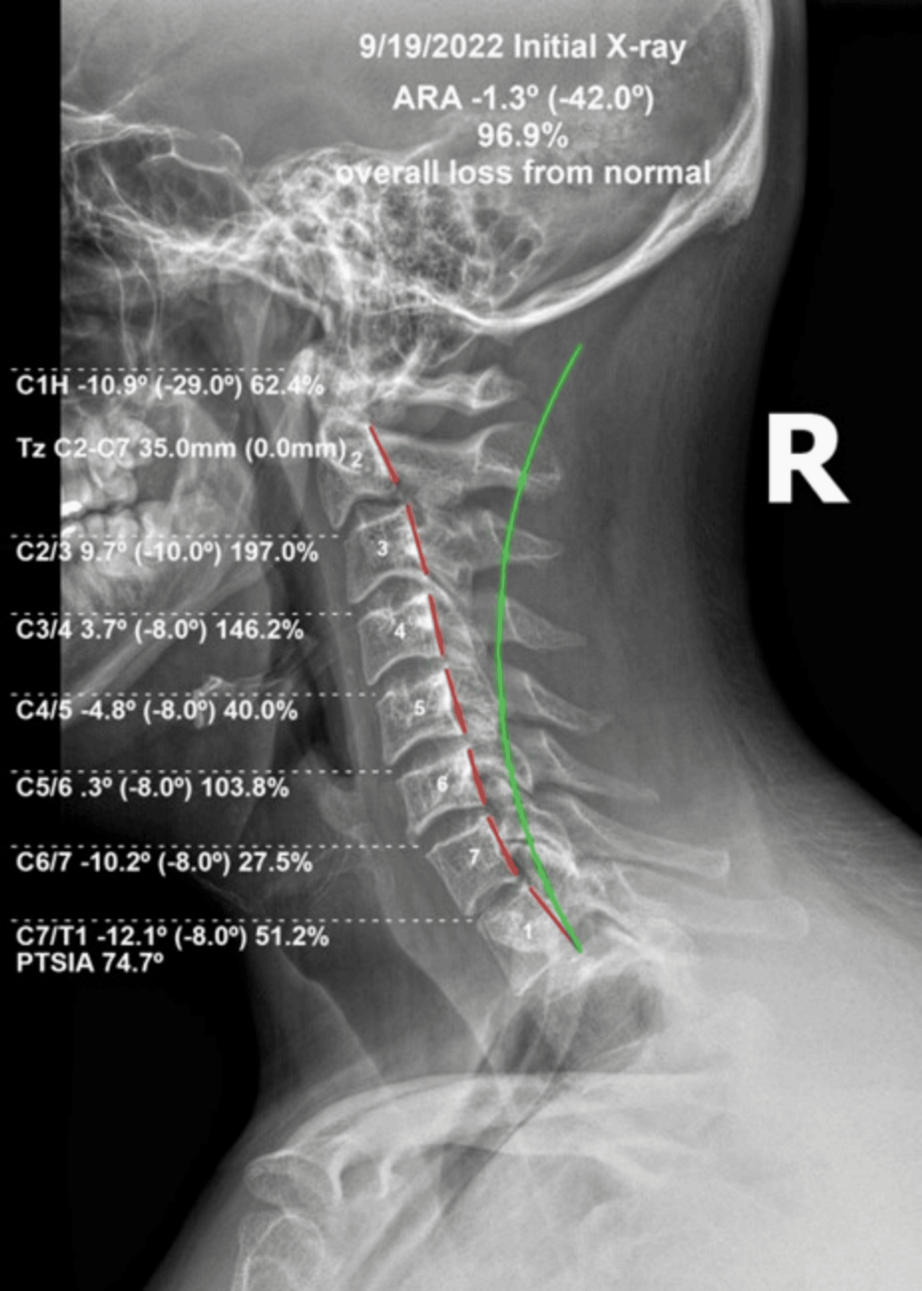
Upright lateral cervical radiograph at the initial examination. The red lines outline the posterior vertebral body margins. The measurements above show the absolute rotation angle (ARA) of -1.3° where a more ideal lordosis would approximate -42° from C2 to C7 (green line). The measurements on the left demonstrate the individual relative rotation of each pair of vertebrae; the patient’s values are listed outside the brackets, and the ideal values are listed inside the brackets.
